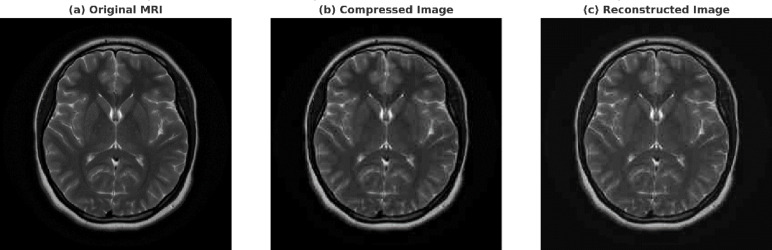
Comparison of MRI images: (a) original scan, (b) compressed version, and (c) reconstructed image using the proposed WT + CAL + VAE-based compression method.
